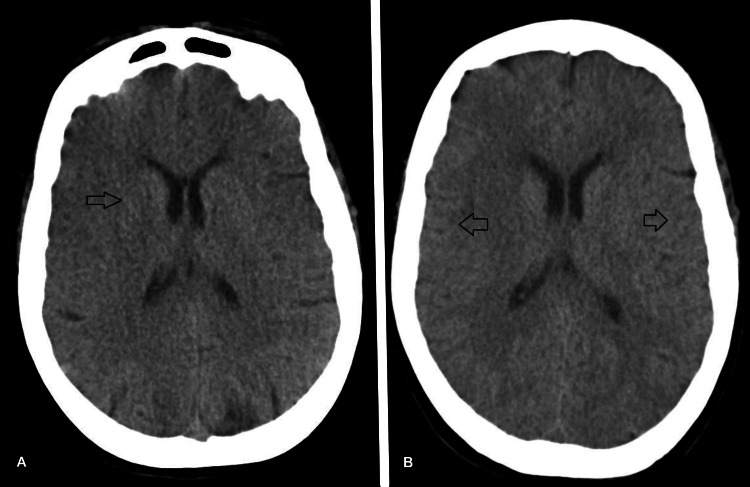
(A) Head CT on day four. (B) Head CT on day 12. Both CT scans show loss of the normal differentiation between cortical gray matter and subcortical white matter. CT: computed tomography
